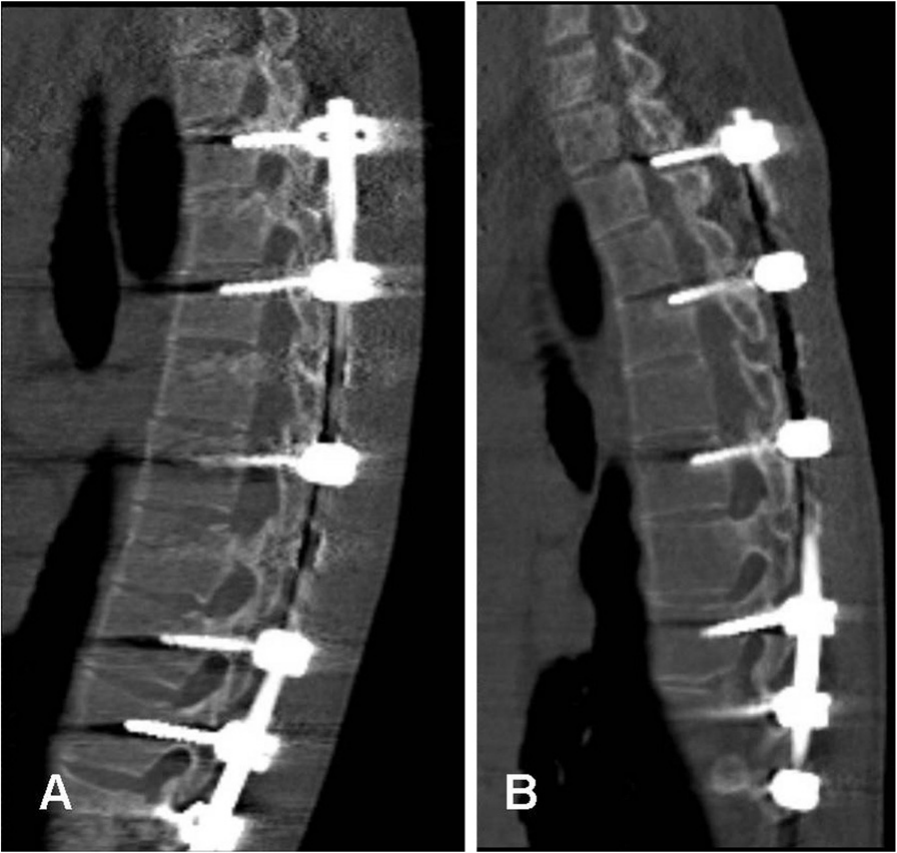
Evidence of screw loosening and pull out. CT of a 16 years old boy following segmental pedicle screw fixation T3-L2: sagittal images of one patient, (A) six weeks following surgery showing normally placed pedicle screws, and (B) two years following surgery showing evidence of pull-out at the level of T3 and T5. The image shows partly evidence of loosening and partly dorsal displacement of the screws and the rod at the upper end of the implant.
